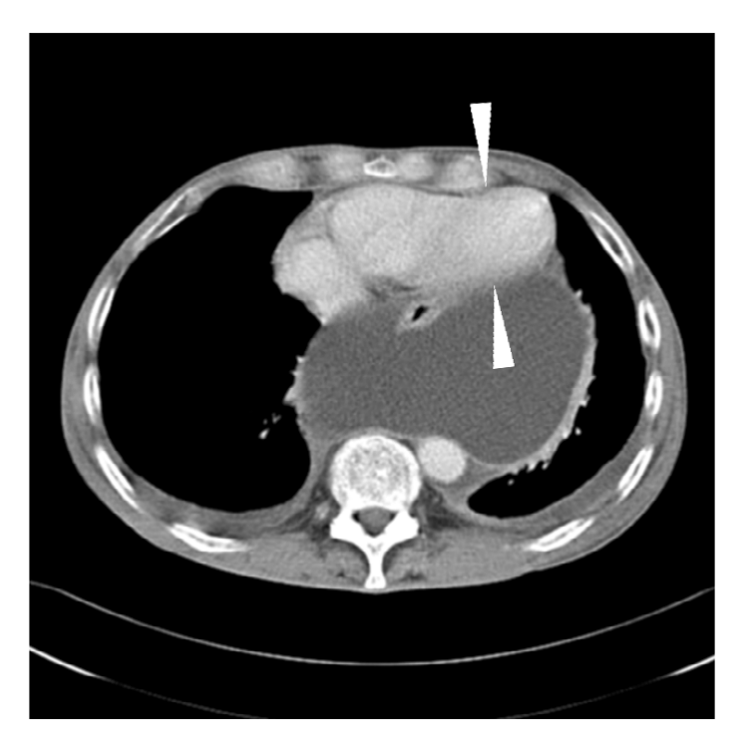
Contrast-enhanced computed tomography scan of the chest-abdomen. A large cystic lesion is compressing the heart, predominantly the left ventricle (arrowheads).
